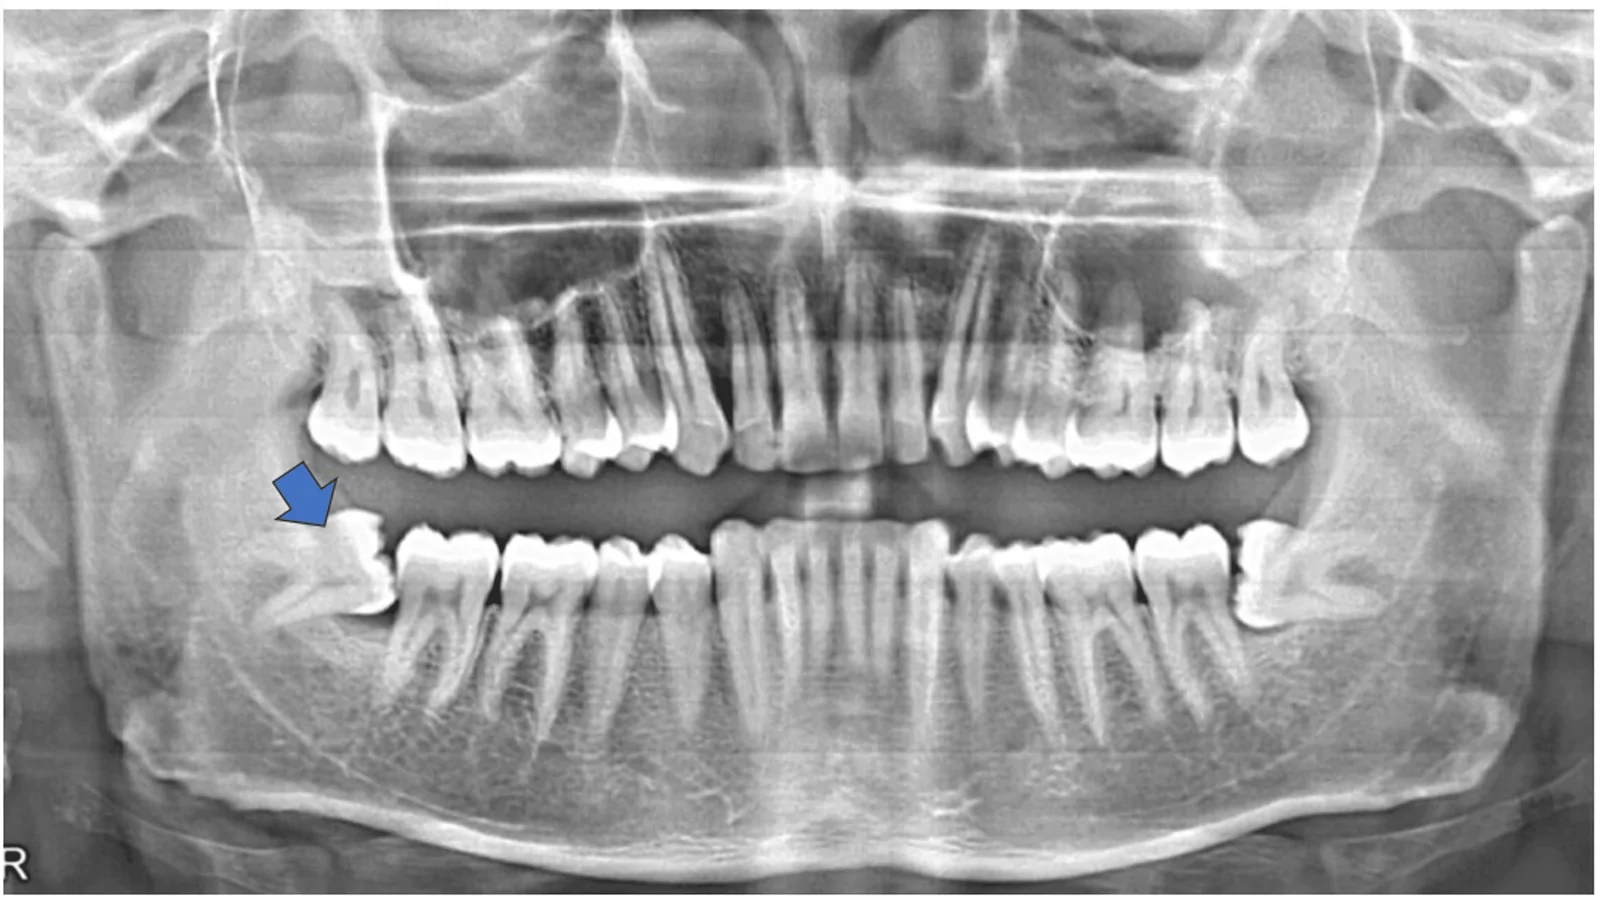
Preoperative panoramic radiograph showing preservation of alveolar bone levels without significant periodontal bone loss. Blue arrow indicates an impacted mandibular third molar (a similar incidental finding was present contralaterally).
